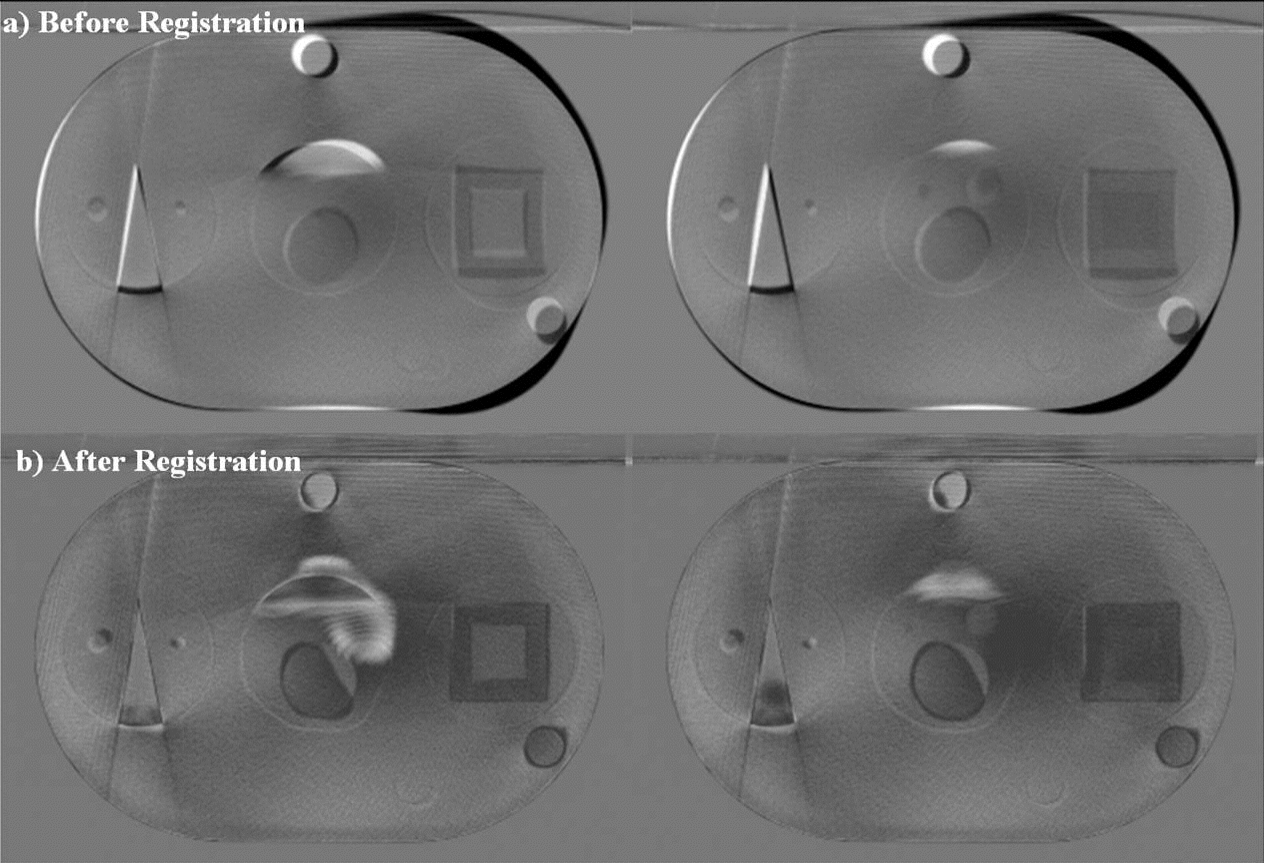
Subtraction images of a cone‐beam computed tomography (CBCT) slice (a) before and (b) after registration to the artificially deformed computed tomography slice with deformations of ±20 mm. These images show the noise present in the CBCT image set.
